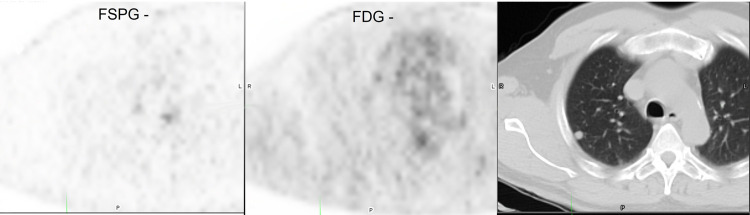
Subject with negative ^18^F-FSPG (SUVmax 0.4) and ^18^F-FDG (SUVmax 0.54) PET/CT.
